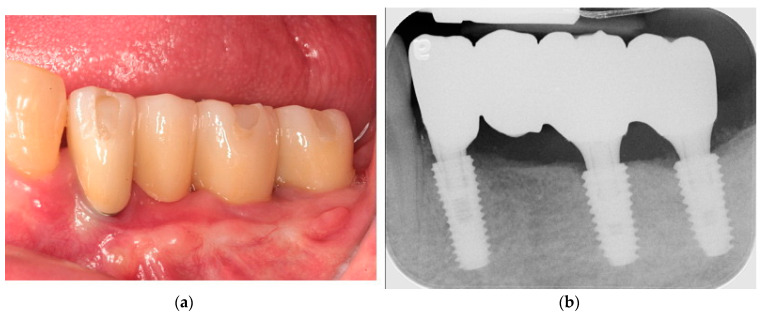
(a) An intraoral image after 2 years of loading. (b) A periapical radiograph indicating stable bone graft over the implant platform after 2 years of loading.
